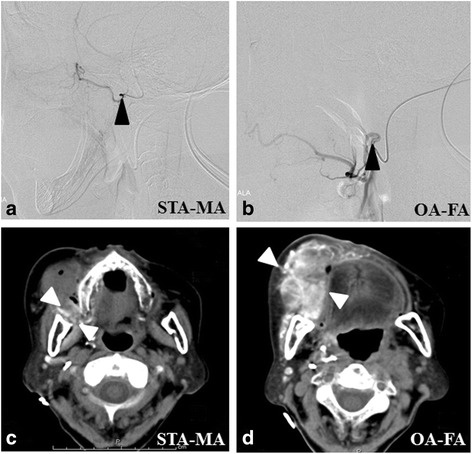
Digital subtraction angiograms (DSAs) and axial views of angio-CT images through retrograde intra-arterial infusion (carcinoma of the right buccal mucosa: T4aN0M0). a, b The catheters are selectively inserted into the right MA via the STA a and into the right FA via the OA b (*black arrow heads*: the tip of catheters). c, d
